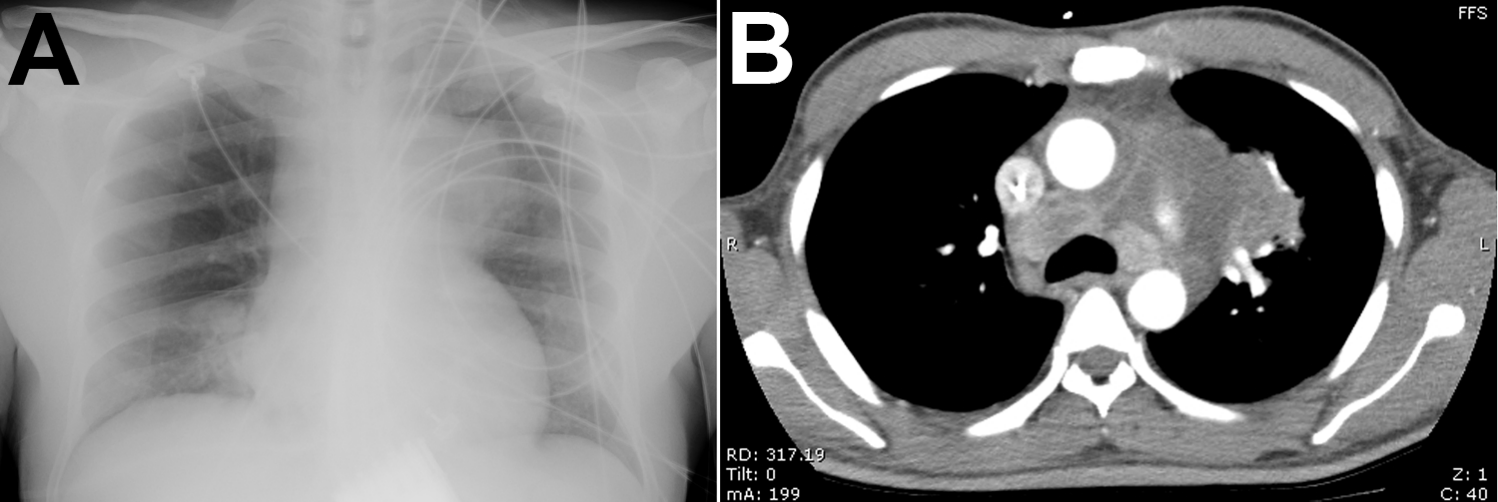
Clinical studies of a patient with melioidosis, Royal Darwin Hospital, Darwin, Northern Territory, Australia. A) Chest radiograph shows a soft-tissue mass associated with the left side of the mediastinum and obscuring the aortic arch. B) Chest computed tomography scan shows a large loculated mass in the anterior mediastinum; the mass is contiguous with multiple enlarged mediastinal lymph nodes and with pulmonary consolidation.
